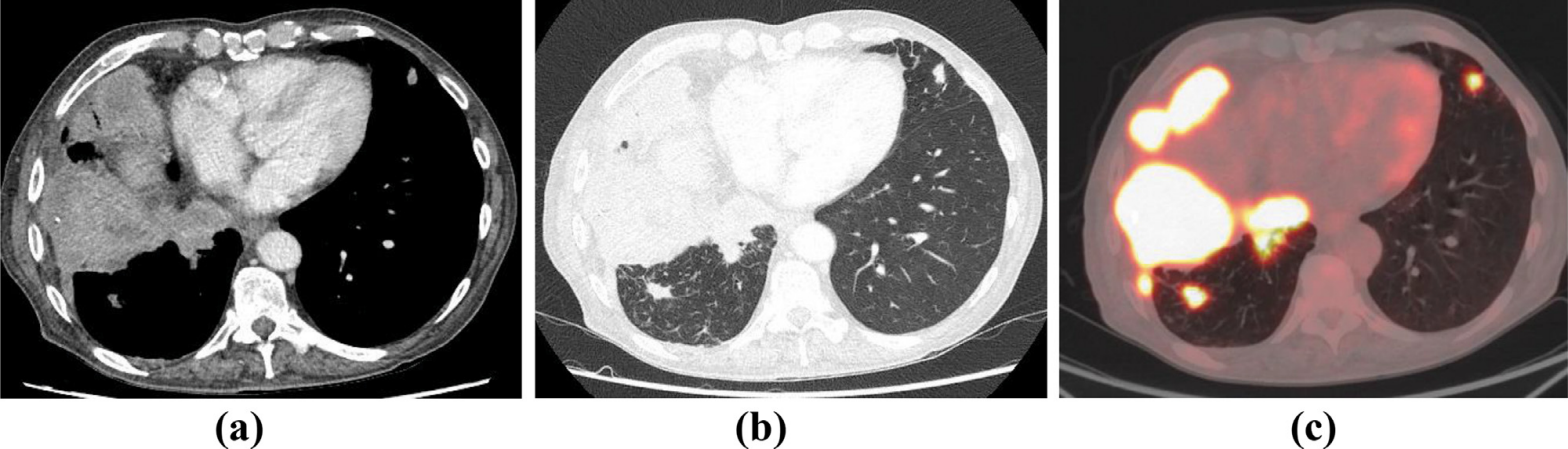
Axial CT images of thorax in (a) mediastinal and (b) lung windows show nodular thickening of right pleura, predominantly over the right hemidiaphragm suggestive of metastatic lung malignancy. Also note volume reduction of right hemithorax due to previous empyema. (c) PET CT axial fused image reveals metabolically active pleural disease. Note FDG avid lung nodule in the lingula. PET complemented CT diagnosis of an aggressive primary pulmonary malignancy.
